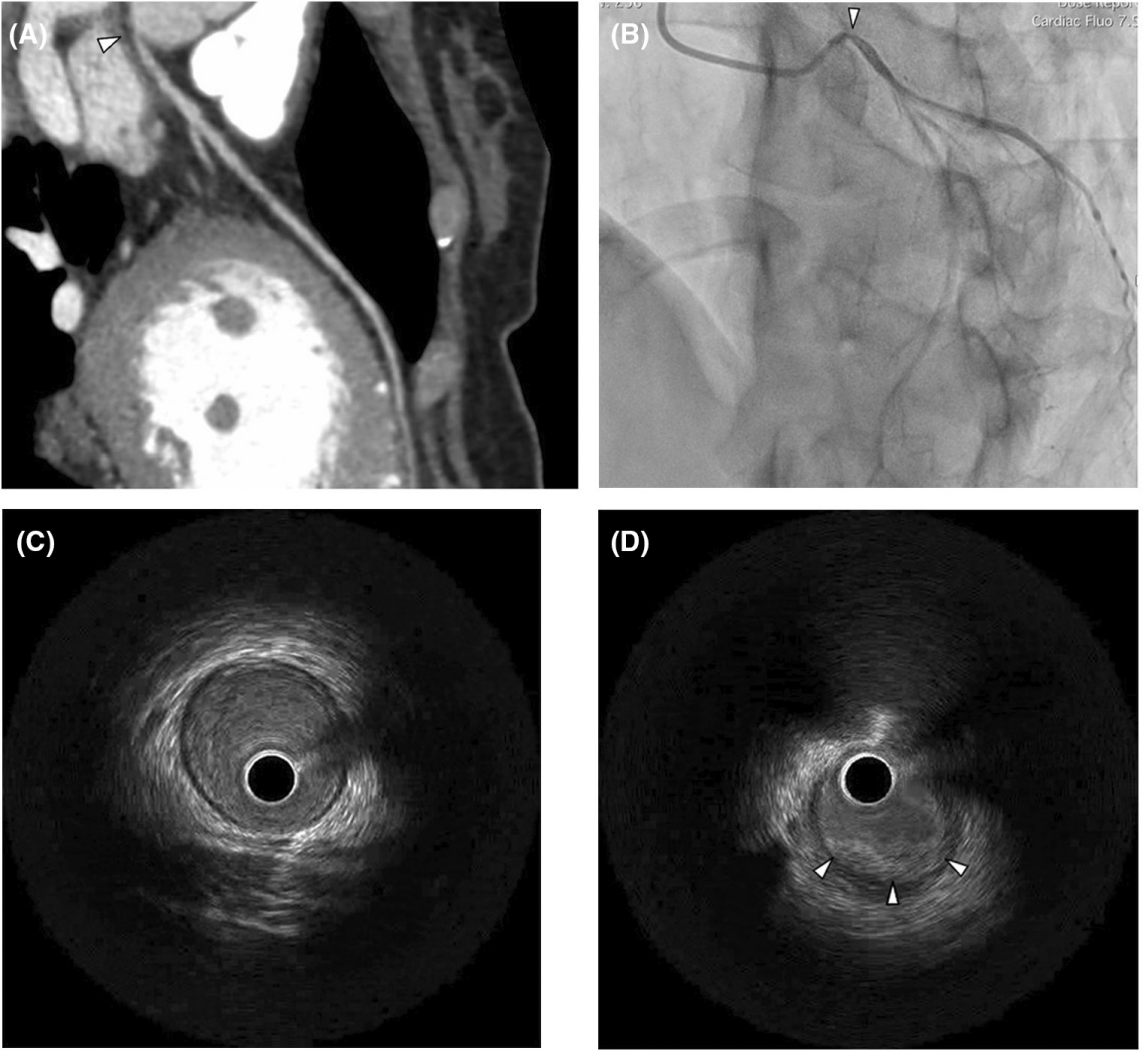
(A) Preoperative multidetector computed tomography showing severe stenosis in the orifice of the left main coronary artery with no evidence of atherosclerotic change (white arrowhead). (B) Preoperative coronary angiography shows severe stenosis of the orifice of the left main coronary artery with no evidence of atherosclerotic change (white arrowhead). (C) Intravascular ultrasound in the non‐stenotic area shows normal intima with adequate diameter. (D) Intravascular ultrasound in the stenotic area shows shrinkage of the wall due to thickened inflamed intima.
